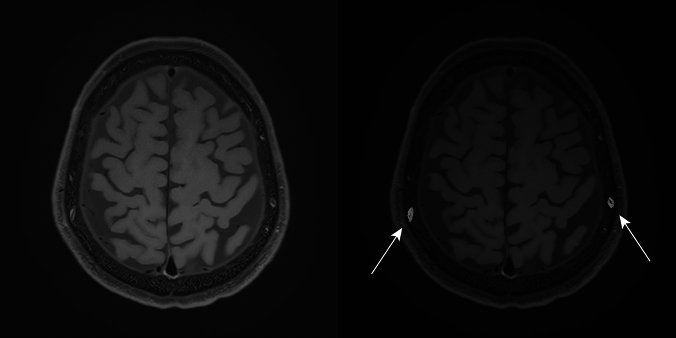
High-signal areas surrounding the diploic veins drawn as regions of interest (*arrows*) on contrast-enhanced motion-sensitized driven equilibrium (MSDE) T1-weighted transverse images
